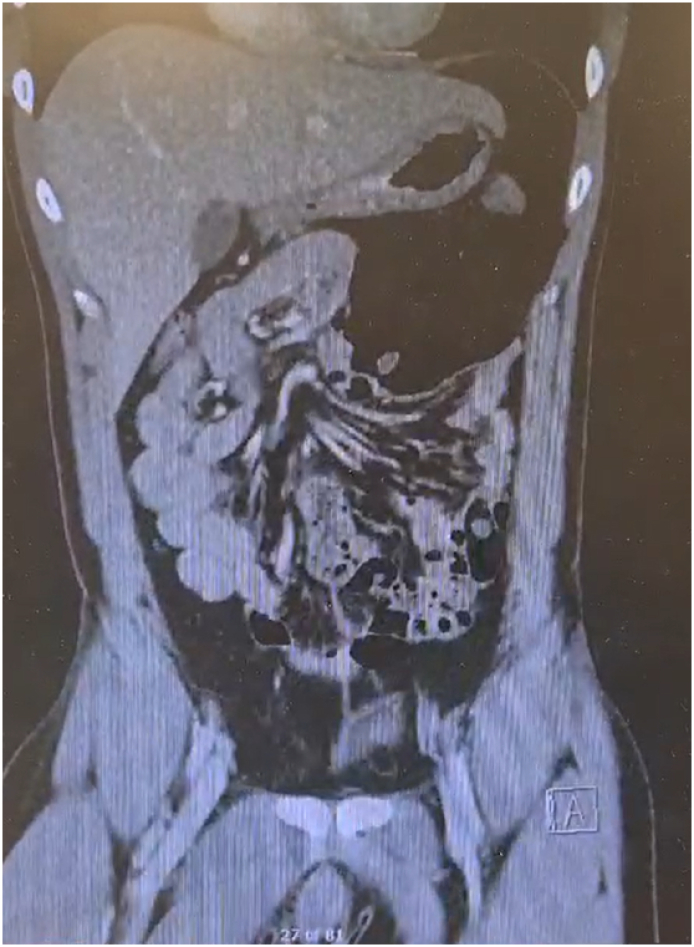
CT abdomen pelvis of Case 3.
